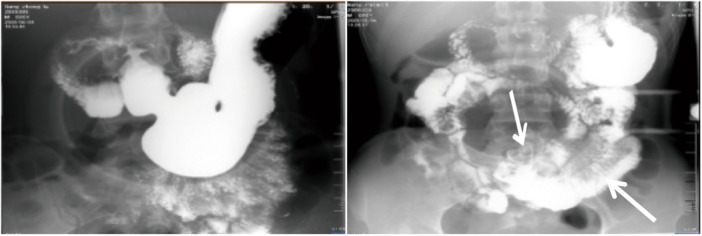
Small‐bowel imaging in Case 2: Multiple segmental strictures. Barium radiograph/enterography demonstrates multiple segmental strictures in the small intestine (white arrows).
